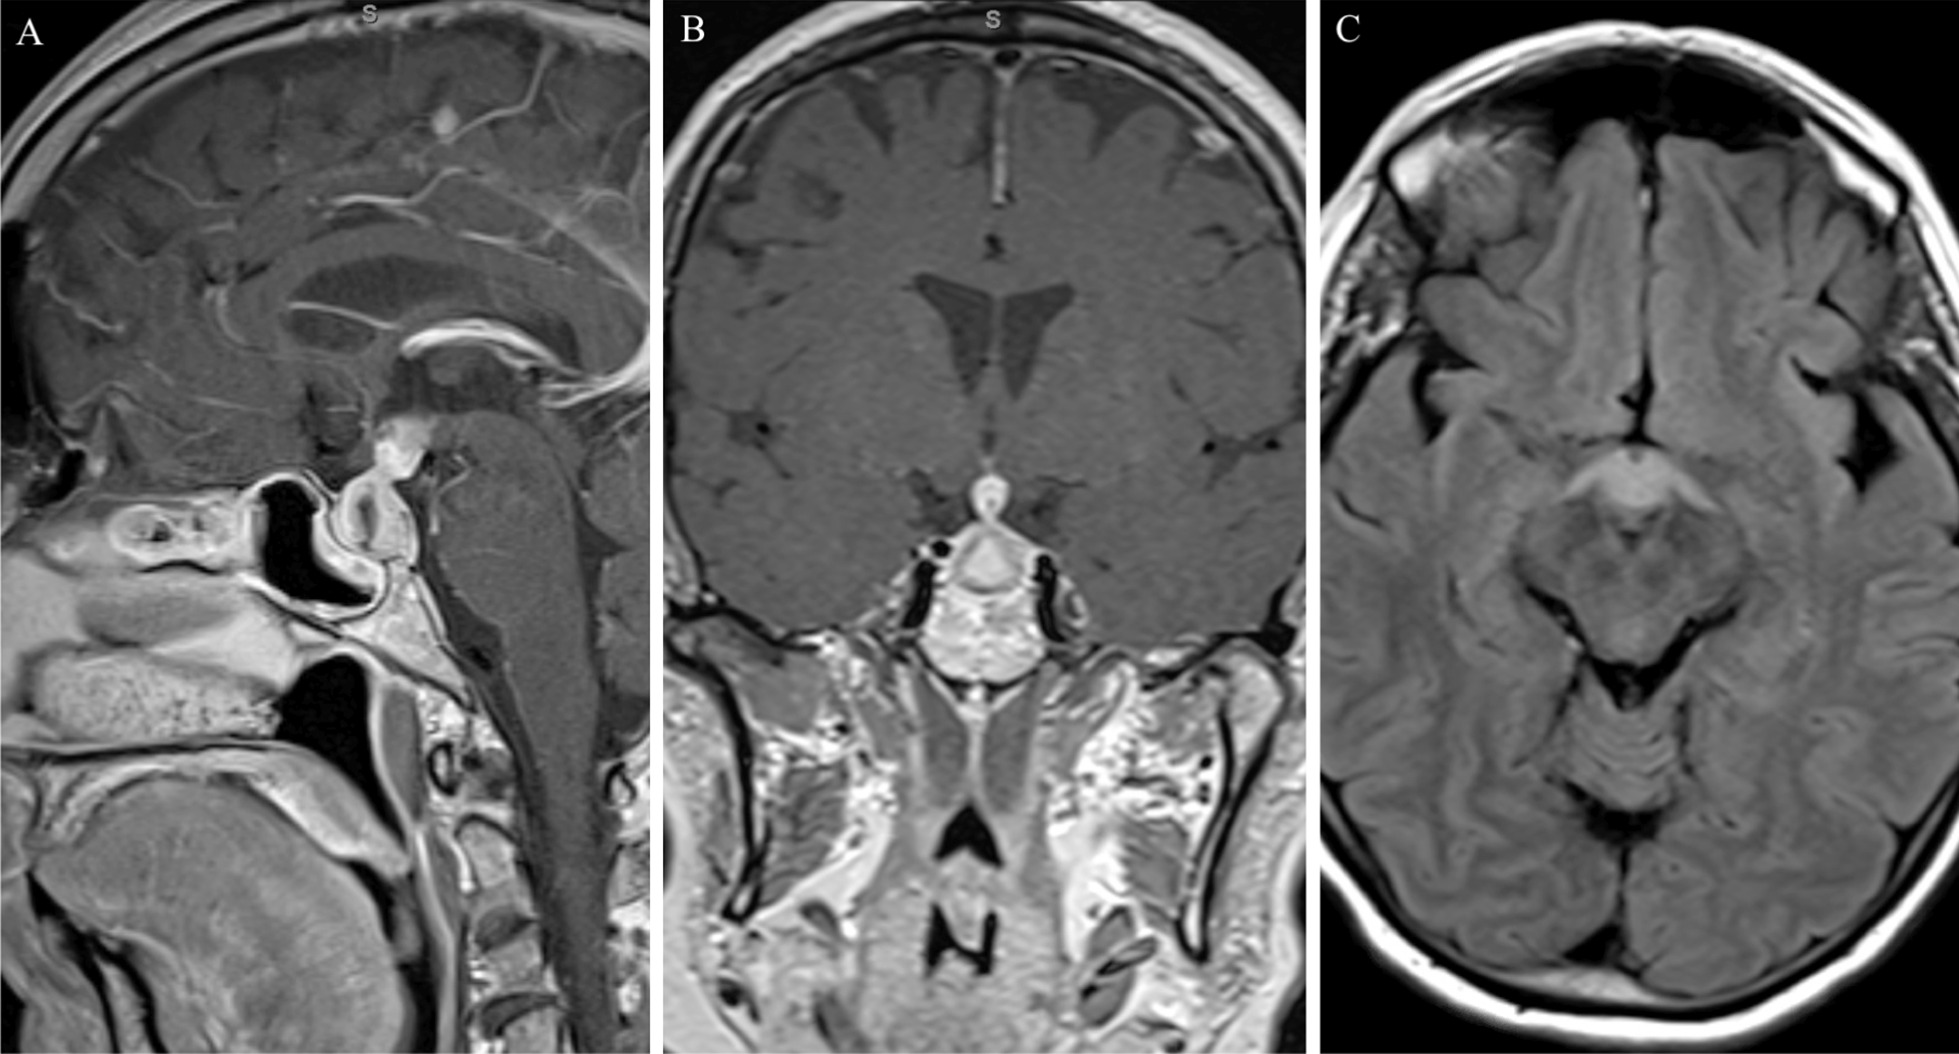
MRI brain with and without gadolinium on initial presentation. Sagittal T1 post-gadolinium (A). Coronal T1 post-gadolinium (B). Axial T2 FLAIR (C)
